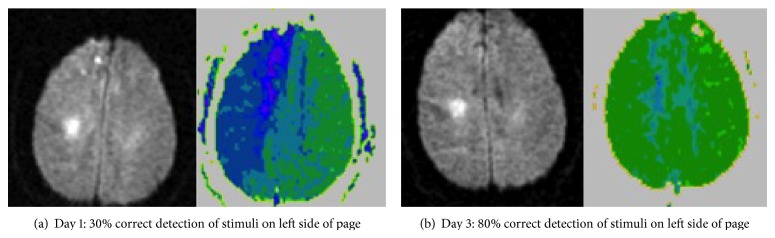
(a) Diffusion-weighted image (DWI; left), showing small subcortical infarct and perfusion-weighted image (PWI; right) of a patient with severe viewer-centered neglect at Day 1. (b) DWI and PWI of the same patient at Day 3, after viewer-centered neglect recovered, as indicated by recovery of detecting stimuli on the left and copying stimuli on the left of a scene. PWI shows that reperfusion of the right frontoparietal cortex was associated with recovery of viewer-centered neglect. In this case, reperfusion was brought about with induced blood pressure elevation.
